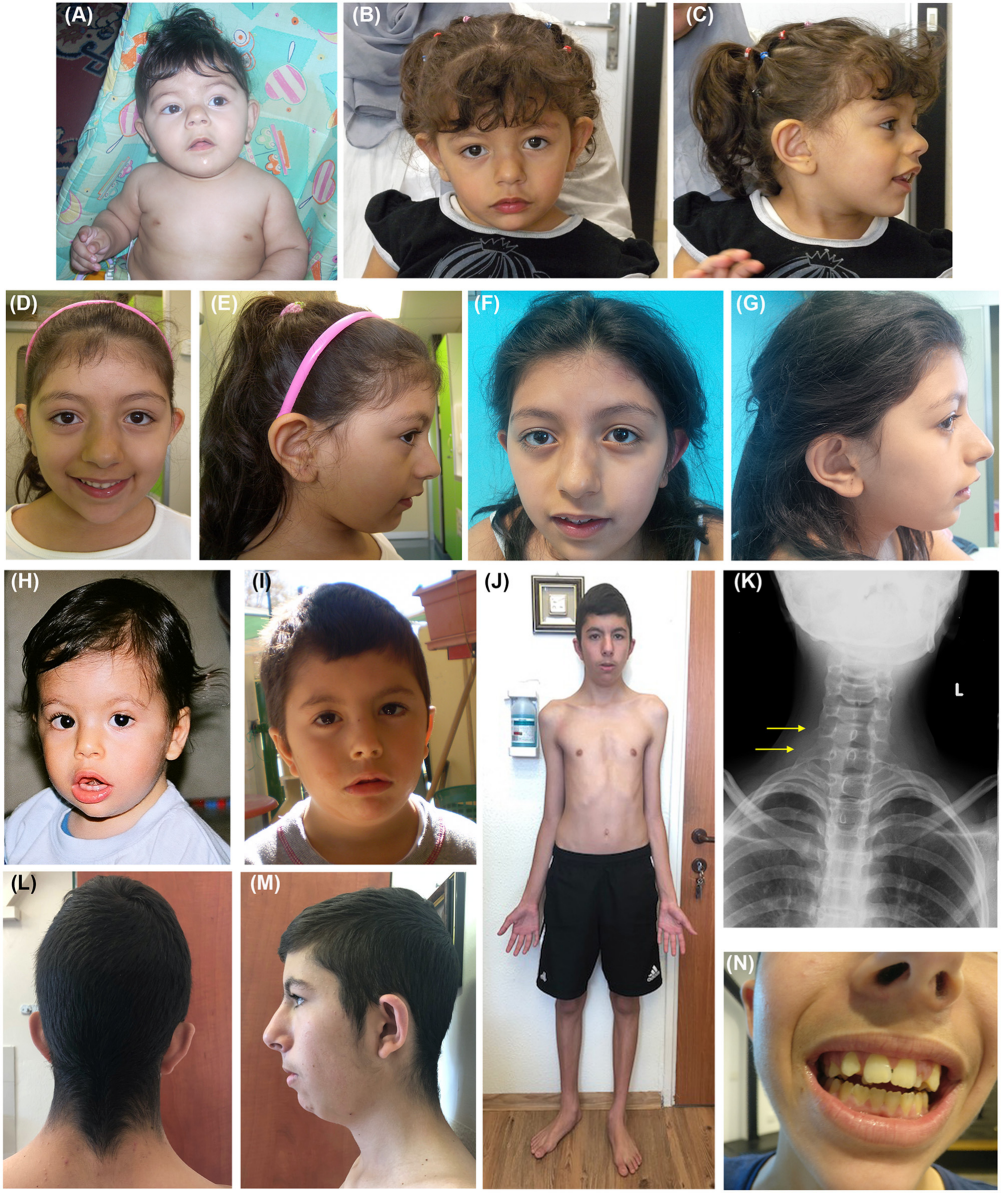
Clinical features of patients 2 and 3. (A) P2 as an infant and at the age of 2 (B,C), 6 (D,E), and 8 (F,G) years. (A–G) Facial dysmorphisms of P2 evolving with age include low anterior hairline, thick eyebrows, long palpebral fissures, and bulbous nose. (H) P3 at 12 months, and at the age of 3 (I) and 16 years (J,L–N). (H–J, L–N) Facial dysmorphisms of P3 evolving with age include triangular face, low anterior, and posterior hairline (L), synophris, long palpebral fissures, anteverted nares, long philtrum, thin upper lip, low set and overfolded ears, overcrowded, and misplaced teeth (N). Other findings such as pectus excavatum, flat foot, and slender habitus can be noticed as well (J). (K) P3 chest radiograph revealing accessory cervical ribs.
